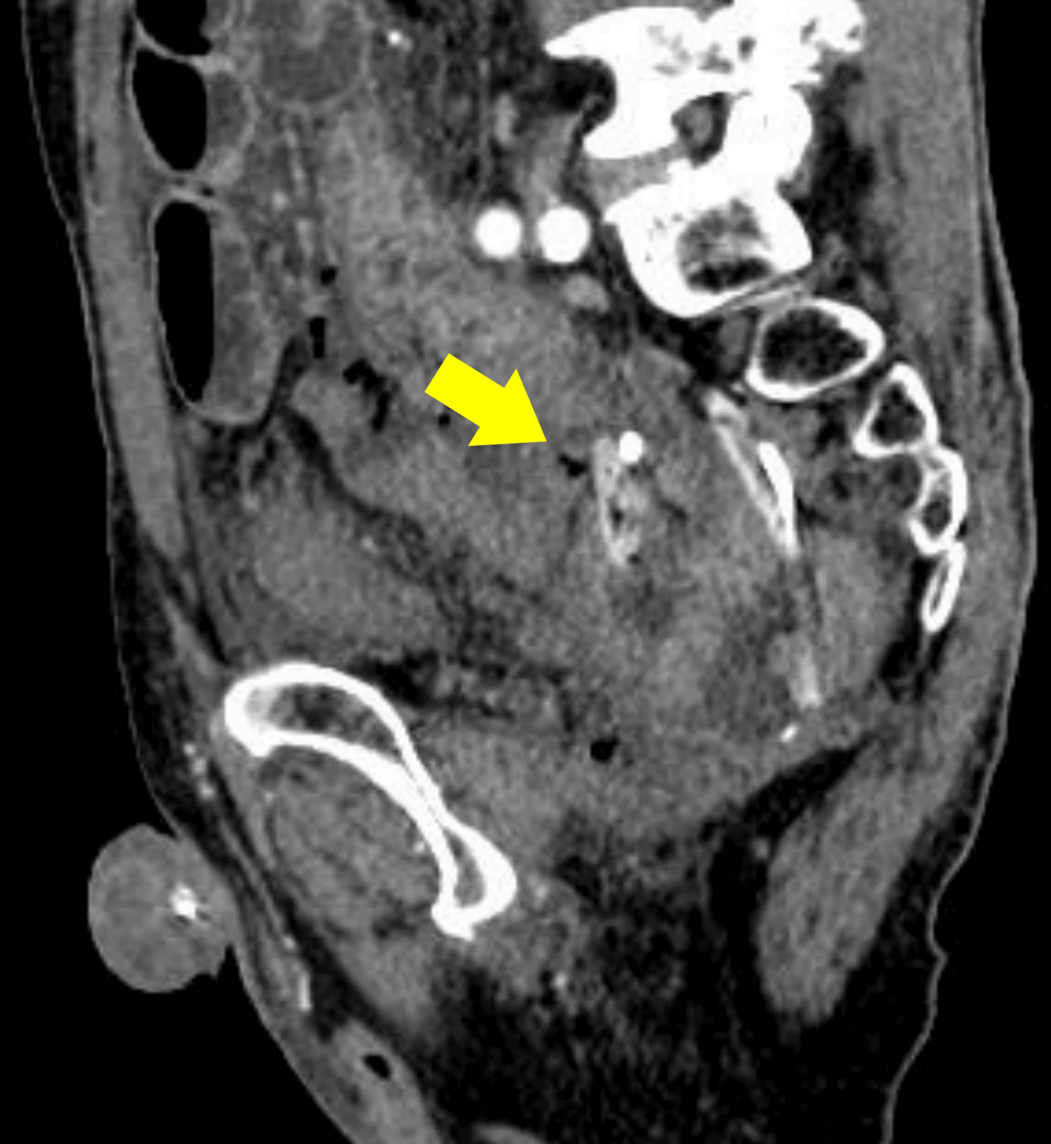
Contrast-enhanced CT image (sagittal view) showing small amounts of fluid and free air around the tip of the transanal drainage tube (yellow arrow), suggesting localized perforation. (CT slice thickness: 1 mm, portal venous phase).
